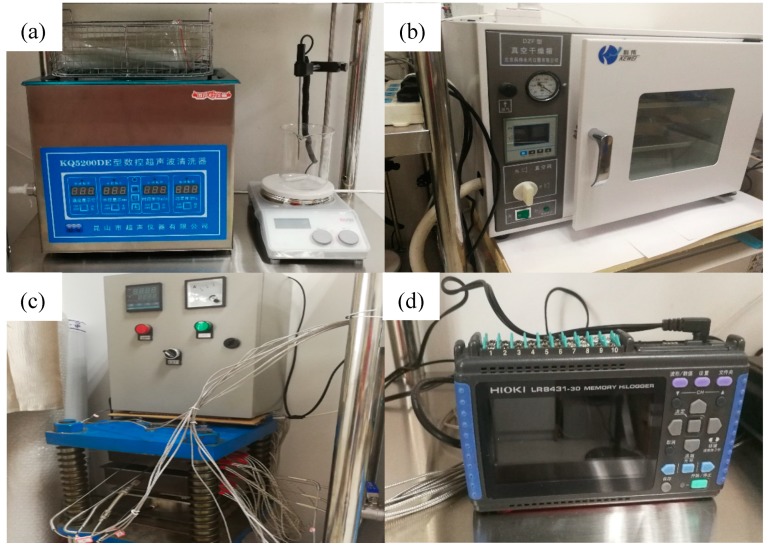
Main instrument and equipment of the vacuum infiltration hot-press forming experimental system: (a) ultrasonic cleaning and magnetic stirrer; (b) vacuum heating infiltration box; (c) hot-press forming and temperature control device; (d) data acquisition instrument.
